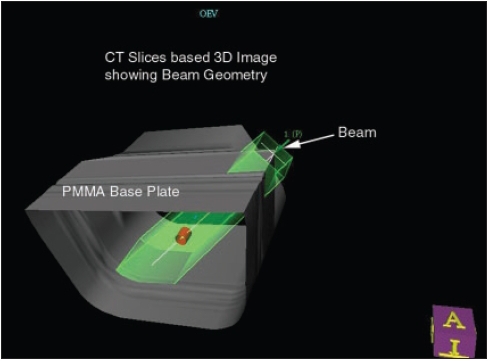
CT based 3D image with beam projection
